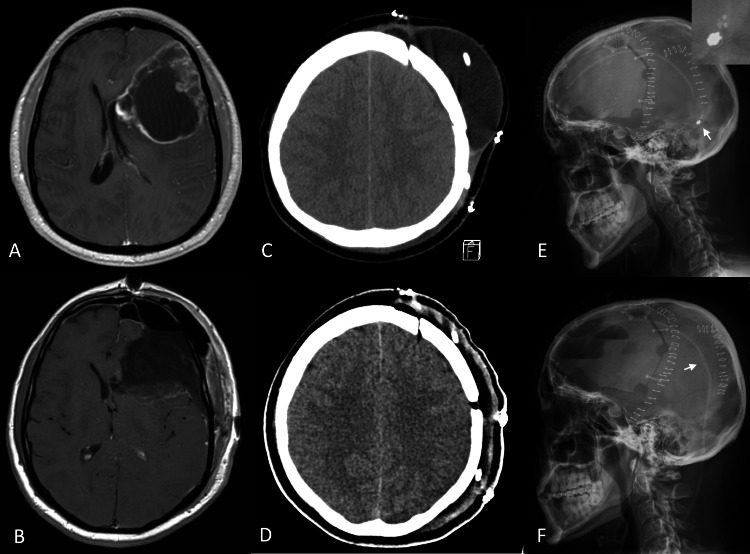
Asymptomatic pseudomeningocele in Case 1 (A) Pre-operative gadolinium-enhanced T1-weighted magnetic resonance imaging (MRI) of the brain revealed a peripherally enhancing mass in the left frontal lobe consistent with a neoplasm. (B) Post-operative gadolinium-enhanced T1-weighted MRI of the brain revealed gross total resection of the lesion (pathology: World Health Organization grade IV glioma). (C) Computed tomography (CT) scan of the head without contrast revealed a large subgaleal pseudomeningocele at the surgical site. (D) CT demonstrating total resolution of the pseudomeningocele at one-week clinic visit after the shunt revision with removal of the shunt valve and direct connection of the proximal and distal catheters. (E) X-ray of the skull demonstrating the Strata II (Medtronic, Minneapolis, Minnesota, U.S.A.) setting at a minimum of 0.5 (white arrow, enlarged figure in right upper inset picture); at this setting, the patient’s pseudomeningocele persisted. (F) Post-revision x-ray of the skull demonstrating proximal and distal tubes connected directly (white arrow); the pseudomeningocele resolved following this revision.
